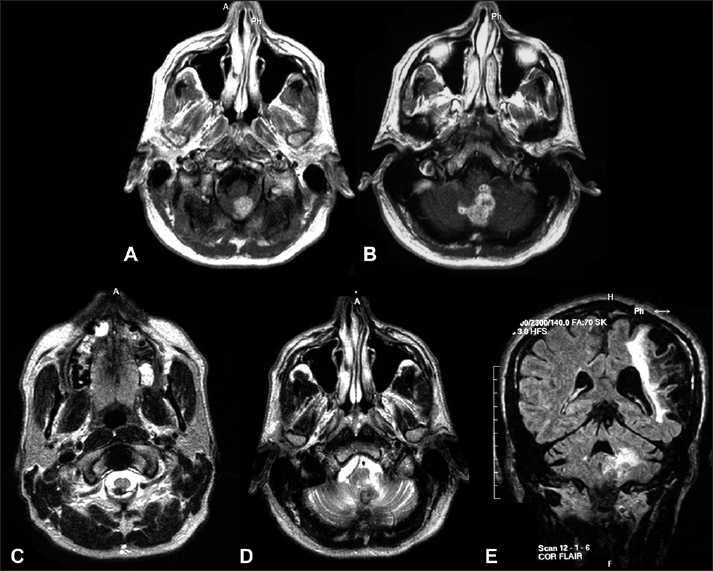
Nine months later, preoperative MRI T1-weighted contrasted axial images showing the enhancing nodular mass in the cerebellar vermis (A) and (B). T2-weighted image showing persistent hypointensity (C) with superior extension (D). Increased FLAIR signal abnormality in the cerebellar hemisphere (E). Before surgery, believed to be neoplastic; at surgery, abnormal tissue with pockets of purulent fluid observed.
